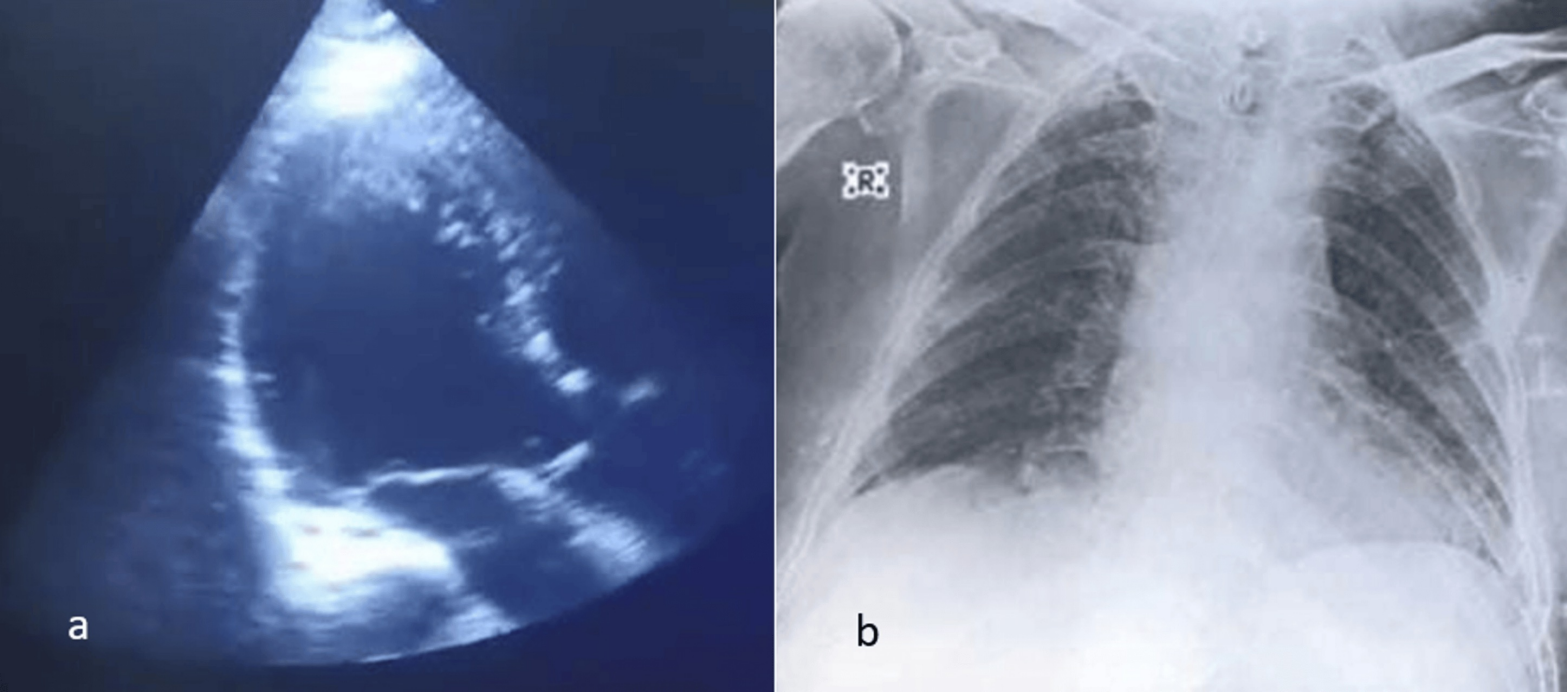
Postoperative images. a. Transthoracic echocardiography showing no pericardial effusion. b. Postoperative X-ray showing a normal heart size
